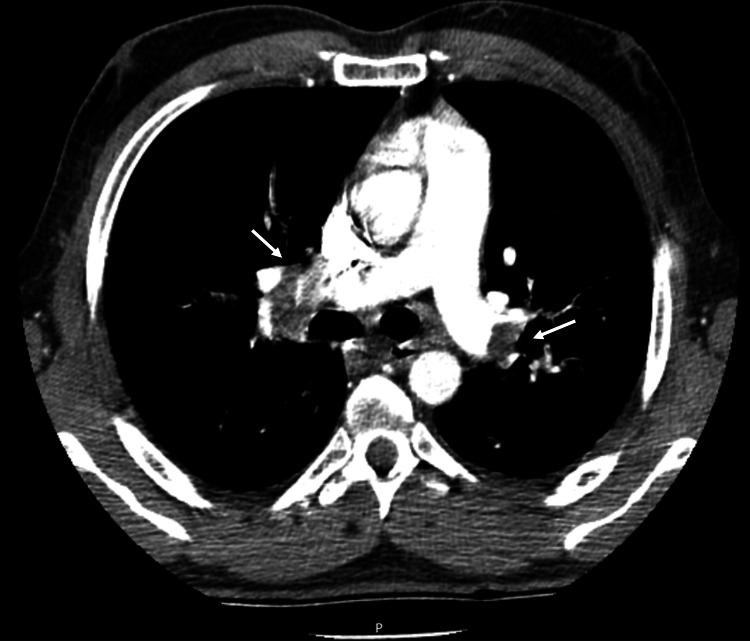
Thoracic contrast-enhanced computed tomography showing extensive pulmonary thromboembolism, with defects at bifurcation sites (white arrows), extending to lobar branches and to the inferior, middle, and lingular segmental branches.
